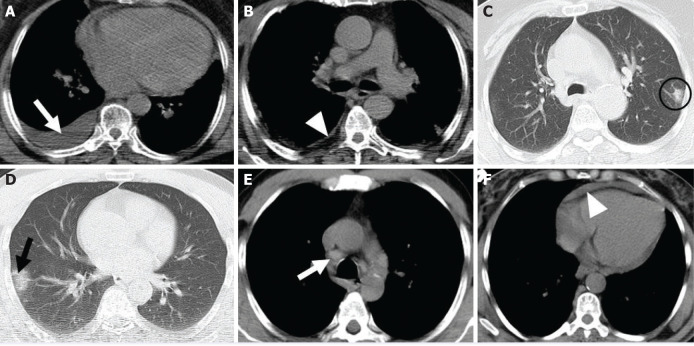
The axial section of the chest computed tomography scan shows atypical findings (A) pleural effusion (white arrow), (B) pleural thickening (white arrow-head), (C) nodule (black circle), (D) reversed halo sign (black arrow), (E) mediastinal lymph nodes (white arrow), (F) pericardial effusion (white arrow-head).
